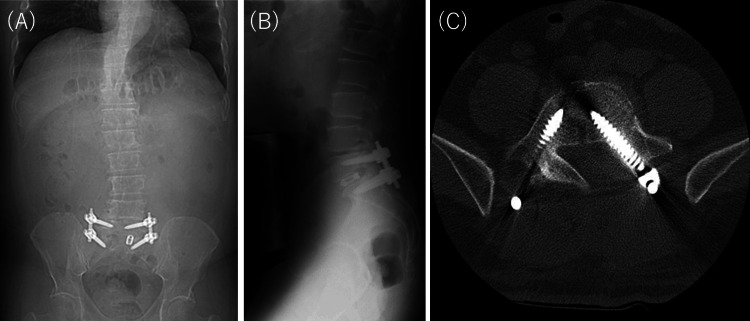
Postoperative lumbar X-rays and CT images CT: computed tomography; TLIF: transforaminal lumbar interbody fusion Postoperative lumbar X-rays and CT images following pedicle screw fixation and TLIF. (A) Anteroposterior radiograph, (B) lateral radiograph, (C) axial CT image
